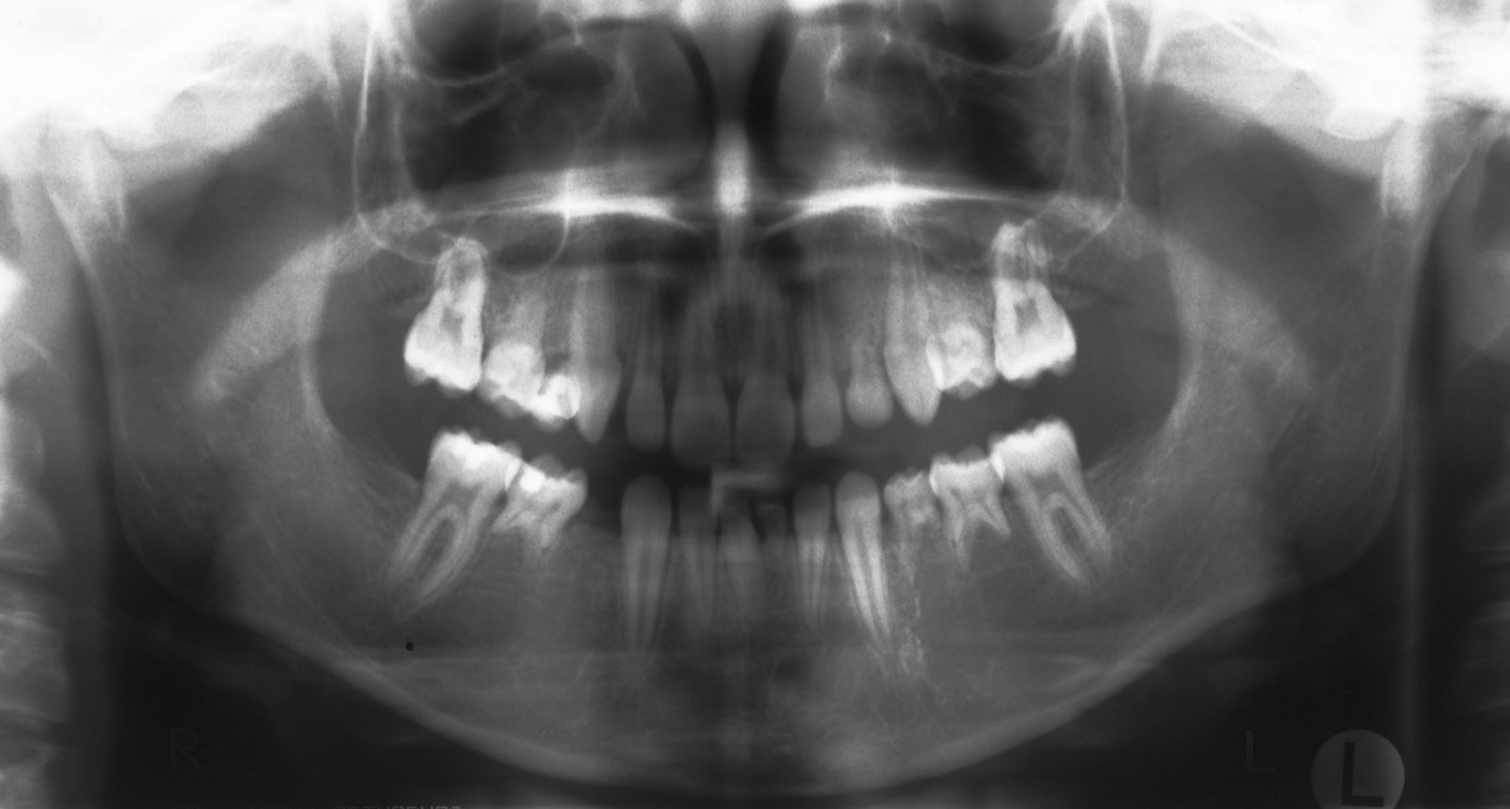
Radiographs of a person with 13 congenital missing teeth: Orthopantomogram.
